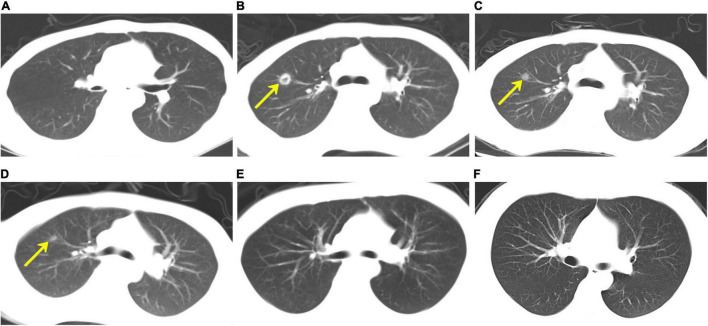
Computed tomography (CT) scan of the chest. (A) As part of his evaluation after haematopoietic stem cell transplantation, chest CT was unremarkable on day + 92. (B) On day + 123, the patient developed a fever and anorexia, and chest CT revealed a new right upper lobe defined nodule (17 × 15 mm) surrounded by a ring of consolidation. Chest CT scans, which were performed on days + 152 (C), + 251 (D), and + 519 (E), respectively, demonstrated a gradual reduction of nodule size. (F) On day + 540, the chest CT revealed that the original right upper lobe nodule disappeared completely.
